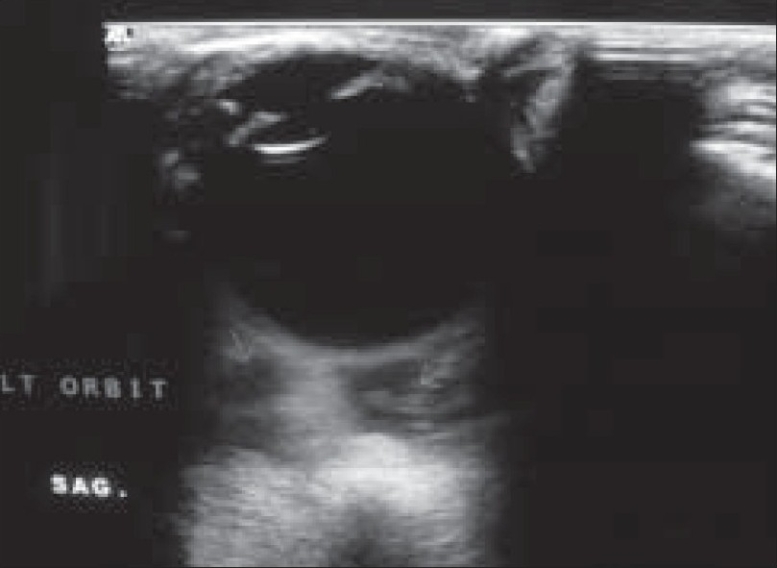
B-mode ultrasonography showed retrobulbar (intraconal) cystic lesions (Arrows)
